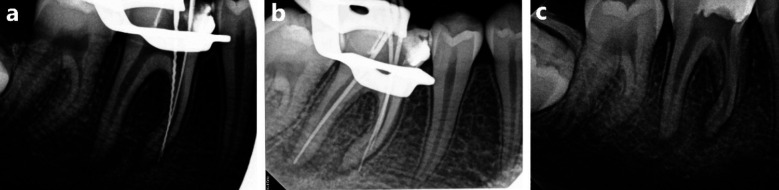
Previous treatment radiographs (a) Showing two files perforating the mesial canals, (b) Showing 2 gutta percha cones perforating the mesial canals, (c) Pre-operative periapical radiograph.
